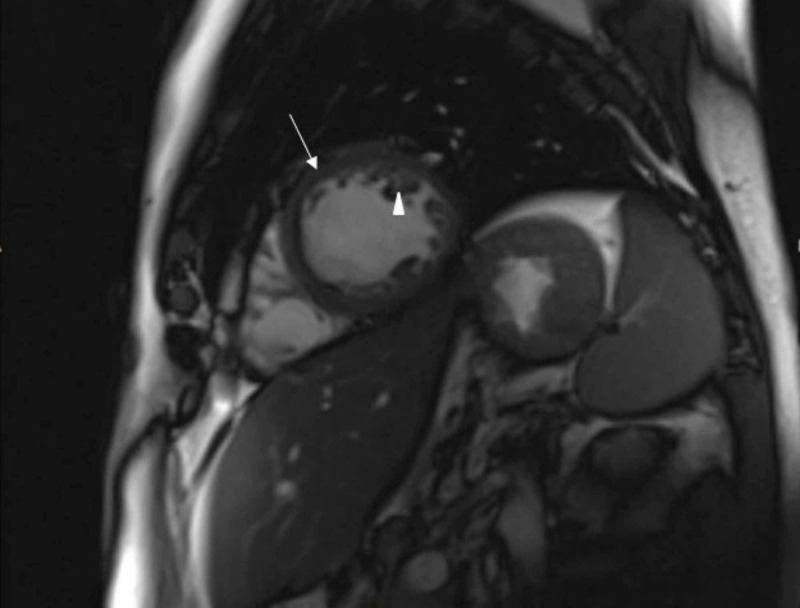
Cardiac MRI showing trabeculations (arrow head), and compacted layer (arrow)
